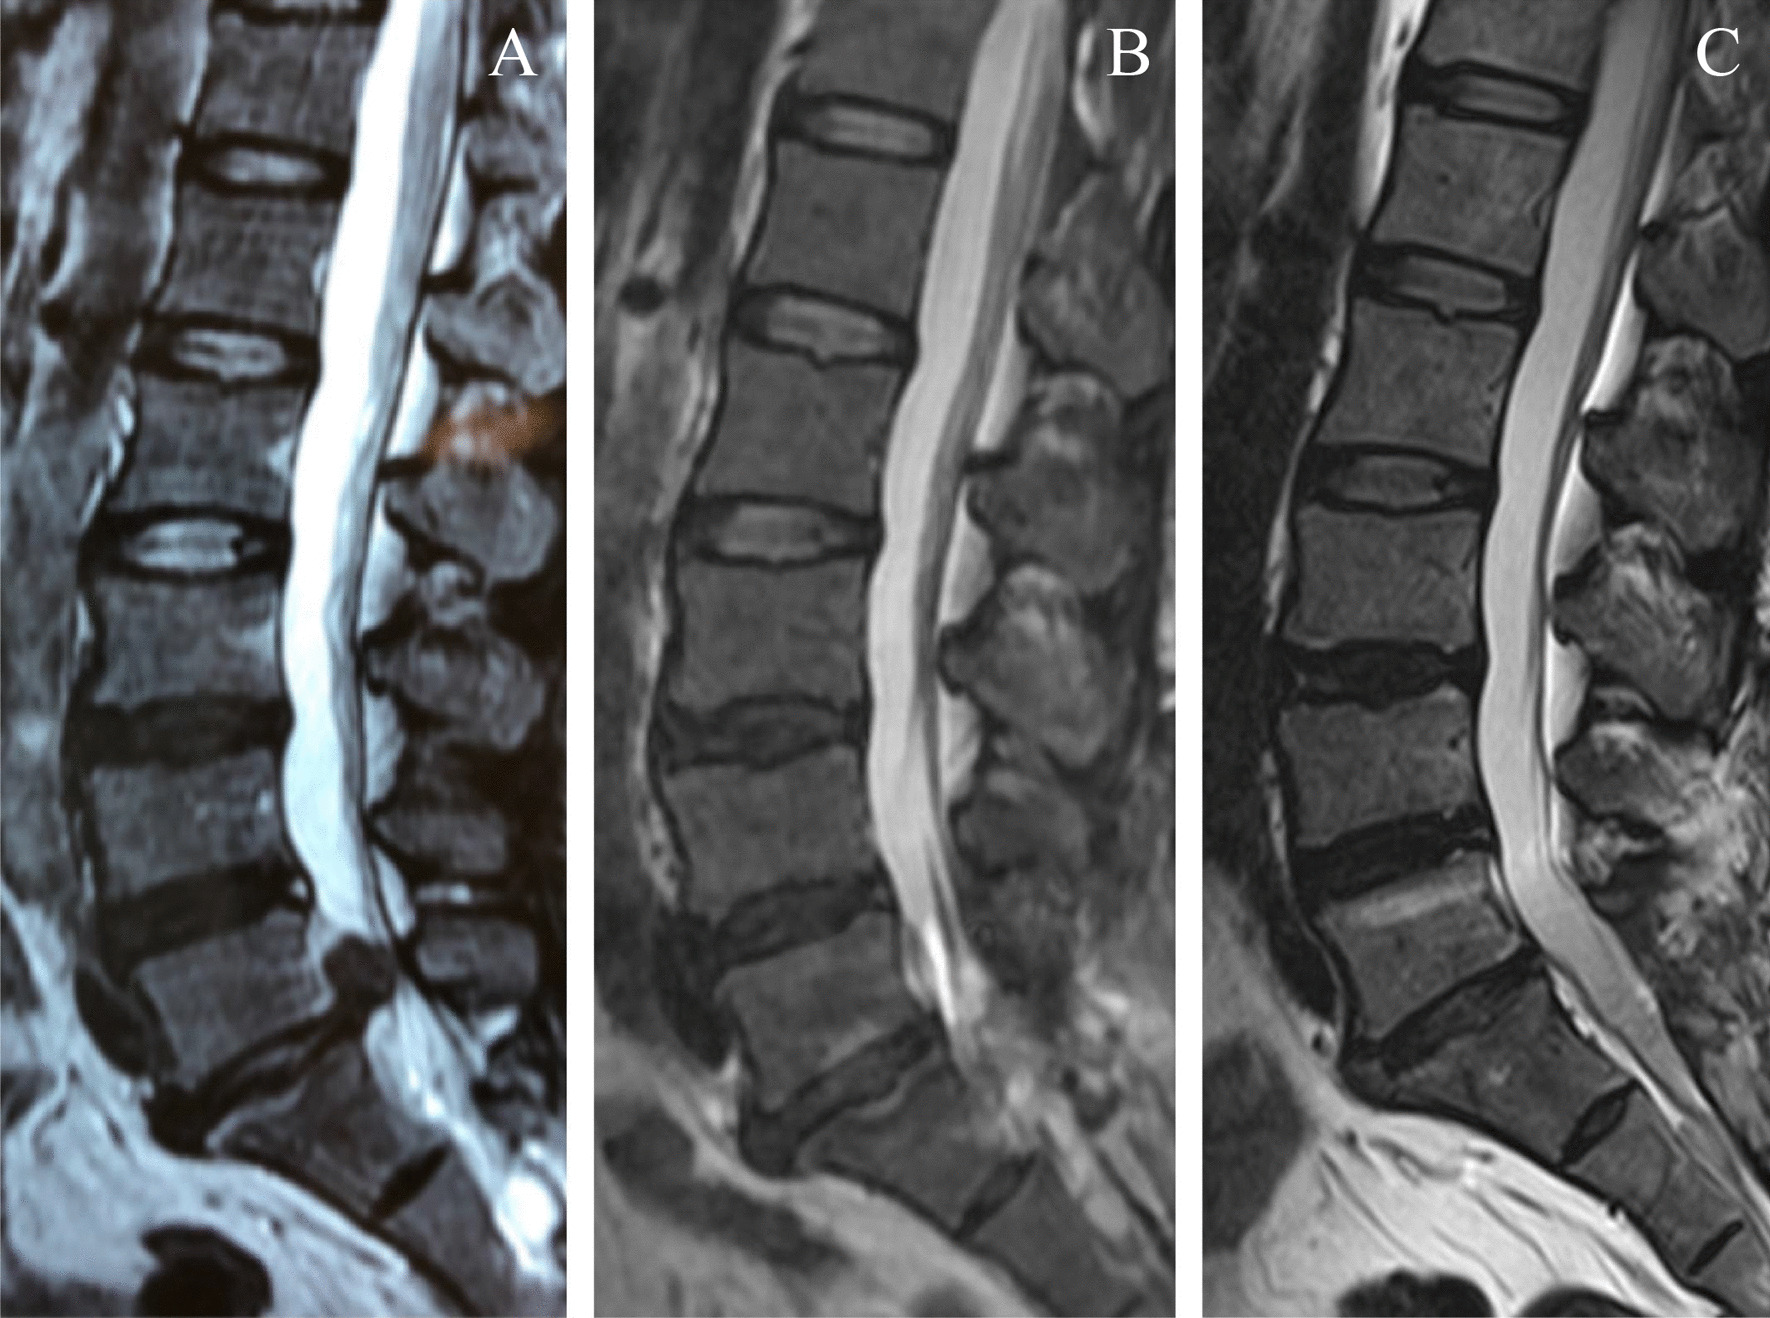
preoperative, 6-month and 12-month sagittal lumbar T2 weighted MRI. A Preoperative sagittal lumbar T2 weighted MRI. Pfirrmann level: 3(L1/2), 3(L2/3), 5(L3/4), 5(L4/5), 6(L5/S1). B 6-month sagittal lumbar T2 weighted MRI. Pfirrmann level: 2(L1/2), 2(L2/3), 5(L3/4), 5(L4/5), 7(L5/S1). C 12-month sagittal lumbar T2 weighted MRI. Pfirrmann level: 4(L1/2), 4(L2/3), 5(L3/4), 5(L4/5), 6(L5/S1)
